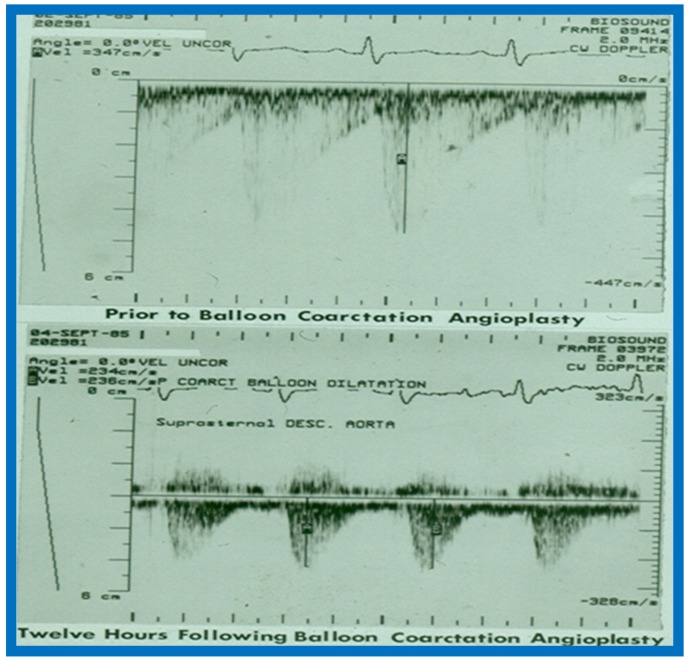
Continuous wave Doppler flow velocity recordings from suprasternal notch view directing the Doppler signal towards the descending aorta prior to (top) and twelve hours following (bottom) balloon angioplasty of aortic coarctation. Note the reduction of peak Doppler flow velocity from 3.47 m/s to 2.35 m/s after balloon angioplasty. Also note that the pandiastolic flow seen prior to angioplasty is no longer seen after angioplasty. Reproduced from Rao P.S. [17].
